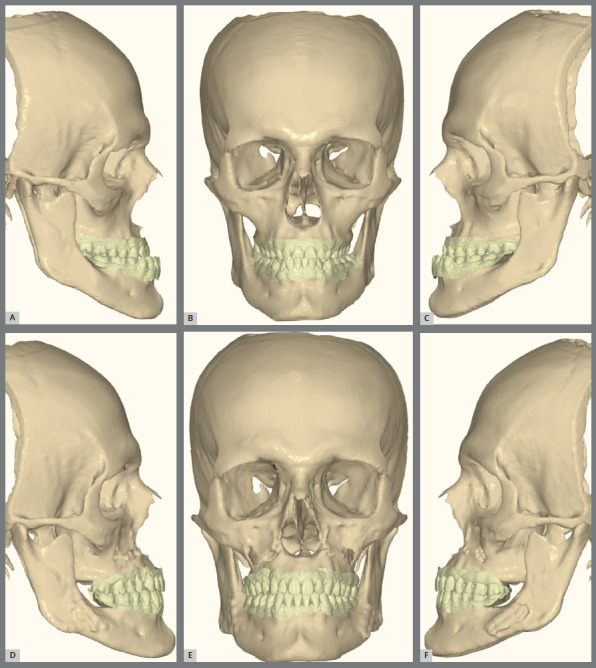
CBCT images: A-C) pre-operative malocclusion, D-F) outcome after orthodontic and surgical treatment.
